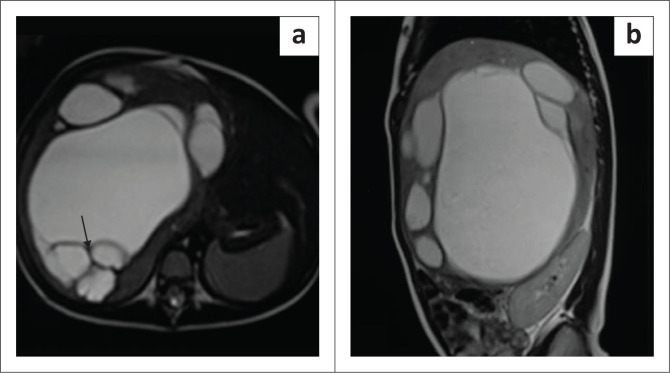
Mesenchymal hamartoma in a 2-year-old female. Axial and sagittal T2-weighted (T2W) MRI images (a and b) of the abdomen showing a multiseptated, hyperintense lesion occupying the right lobe of liver with internal hypointense septae (arrow in a). The lesion is indistinguishable from a hydatid cyst on imaging.
